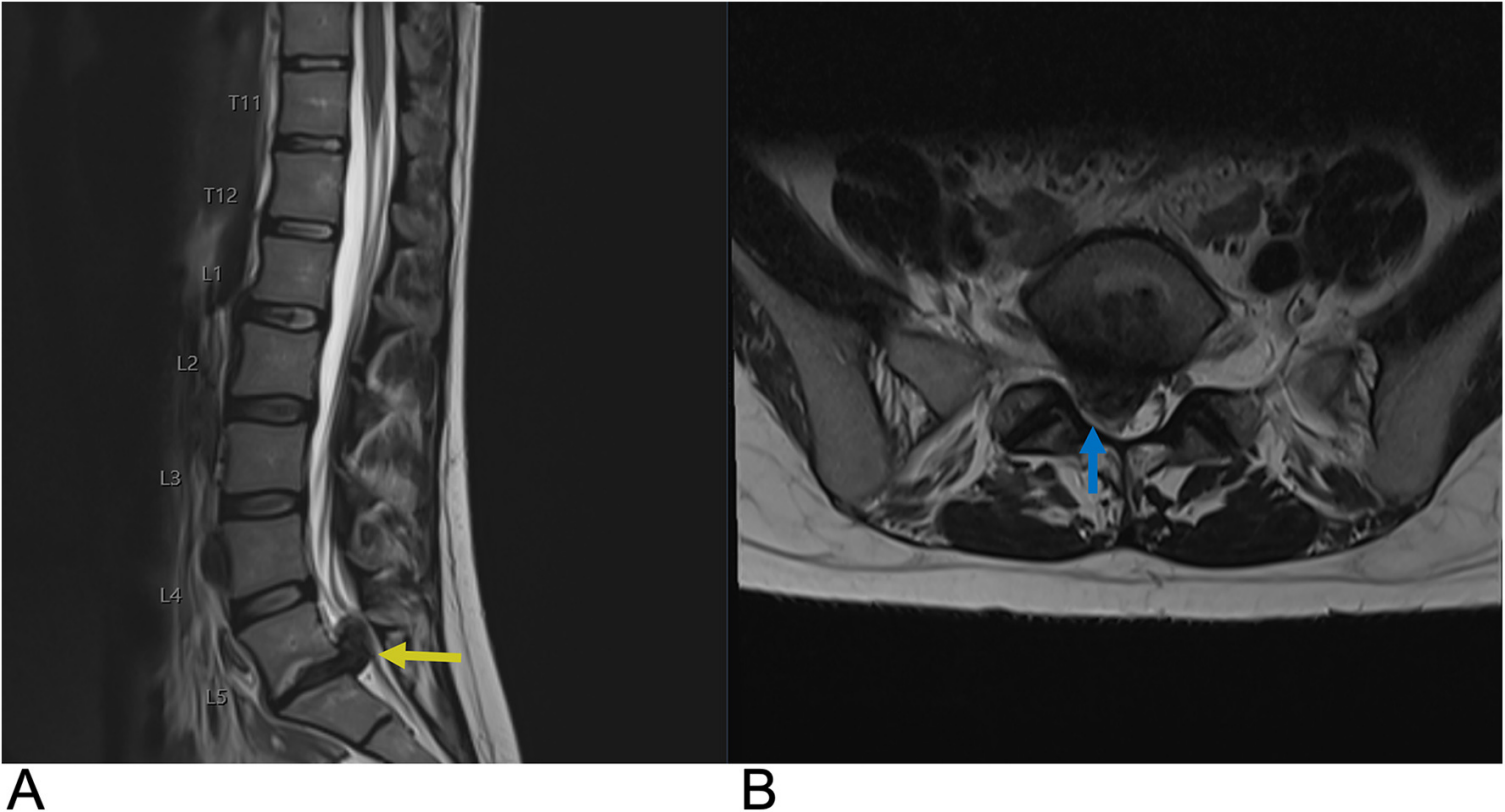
Disc herniation. Sagittal T2 (A) and axial T2 (B) MRI of the lumbar spine demonstrating a large right paracentral broad-based disc herniation at the L5-S1 level (yellow arrow) with the herniated disc pushing into the spinal canal. On axial MRI, the herniated disc can be seen causing effacement of the right subarticular zone with compression of the right S1 nerve root (blue arrow).
